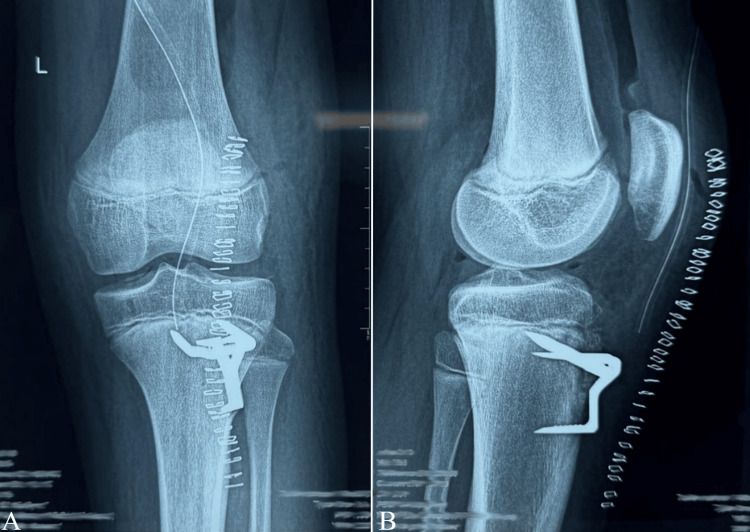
Postoperative radiographs of the left knee one day after the surgical fixation of the tibial tubercle using staples, in anteroposterior (A) and lateral (B) views
